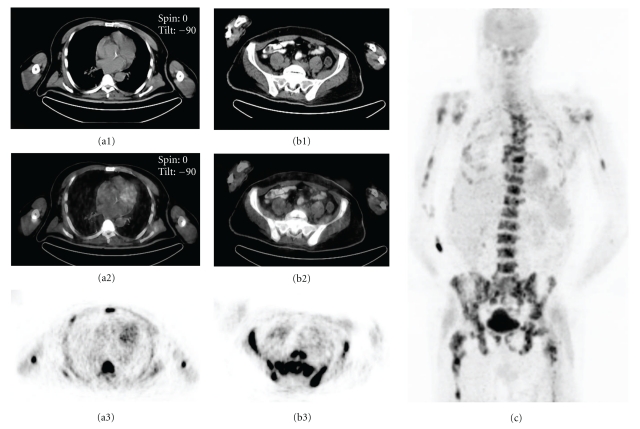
A 58-year-old man with long standing FUO. Diffuse FDG accumulation in bone marrow with no extraosseous manifestations was shown on the transaxial slices of CT (a1, b1), fusion (a2, b2), and PET (a3, b3) and anterior MIP image (c). Non-Hodgkin's lymphoma was diagnosed by bone marrow biopsy.
